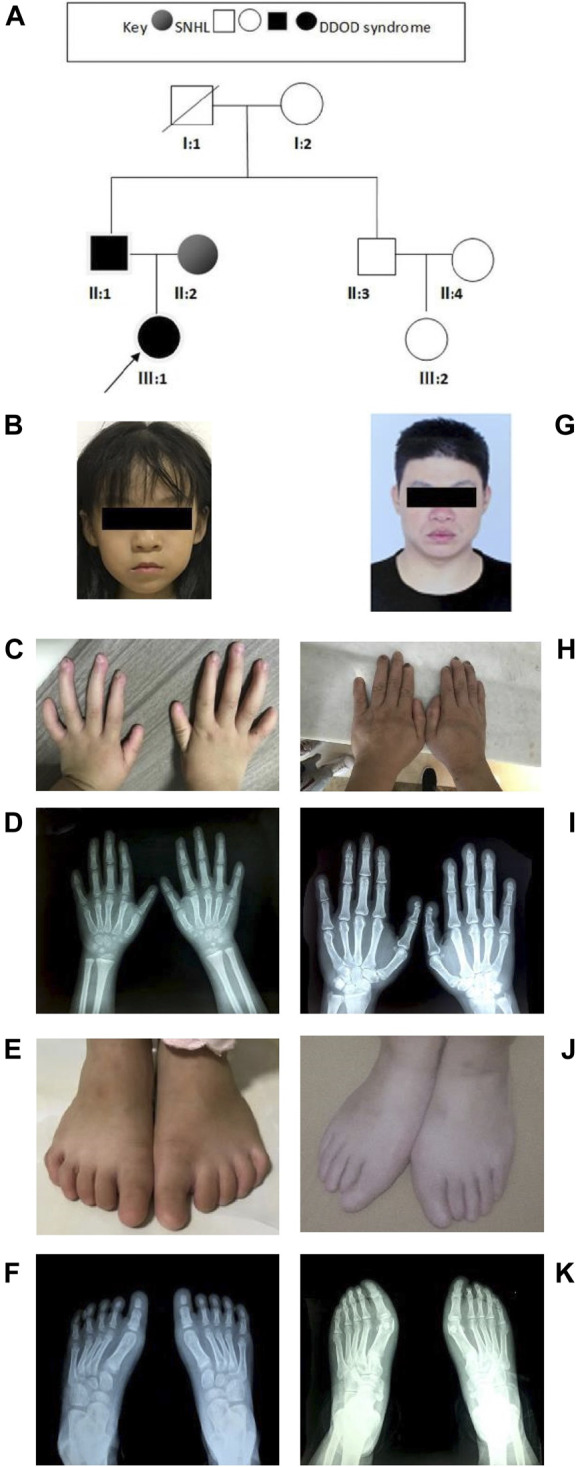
Clinical findings of family 2. (A) Family tree. (B–F) Proband and onychodystrophy. Absent fingernails on the first- and fifth-digits hypoplastic fingernails. Toenails are totally absent. Hand and foot X-rays show normal phalanx development. (G–K) Phenotypes of onychodystrophy of the father are similar to the proband.
